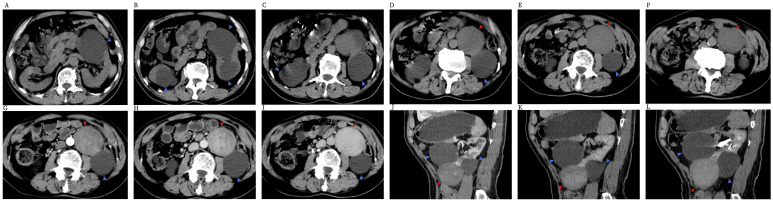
(A–F) Abdominal unenhanced scan CT. Blue arrows indicate cysts; red arrows indicate masses. Multiple cysts are visible in both kidneys. A solid, uniform mass is observed anterior to a cyst in the lower pole of the left kidney, with a CT value of approximately 37 HU. The lesion has a regular morphology and well-defined margins. (G–I) Abdominal contrast-enhanced CT images. (G) Mild heterogeneous enhancement of the lesion is seen in the arterial phase. (H) In the portal phase, the lesion shows progressive enhancement (CT value: 93 HU). (I) In the delay phase, the lesion shows gradual enhancement (CT value: 95 HU). (J–L) Sagittal CT images: (J) arterial phase, (K) portal phase, (L) delay phase. The lesion is located between two giant cysts in the left kidney and is connected to its lower pole. The contrast-enhanced scan shows mild, gradual enhancement of the lesion.
